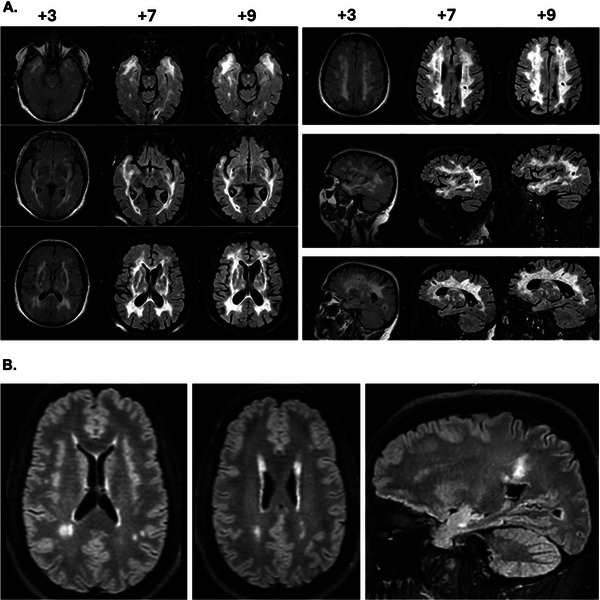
(A) Brain MRIs (FLAIR sequences, axial and sagittal sections) of the index patient (Patient #1) in different time periods (3, 7, and 9 years after the initial episode of neurological dysfunction). There is WMH of presumed vascular origin, which includes the anterior temporal lobes and external capsules, and evolves over time in consecutive MRI scans. The patient was found to harbor the p.Cys206Trp *NOTCH3* variant. (B) MRI (FLAIR sequences, axial and sagittal sections) of the index patient's son (Patient #2) carrying the p.Cys206Trp *NOTCH3* variant. A brain MRI scan showed WMH of presumed vascular origin, of similar nature but lesser extent than that of his mother (Patient #1). Abbreviations: WMH, White Matter Hyperintensities.
